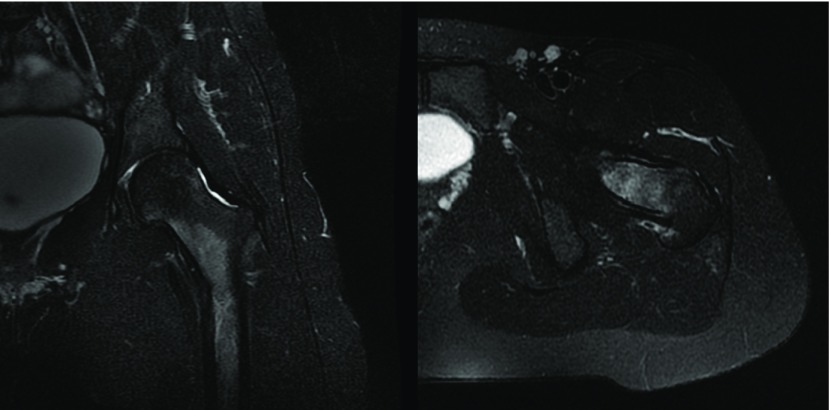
MRI of the left hip, STIR sequence, showing hyperintense signal, characteristic of a compression type femoral neck stress fracture.
